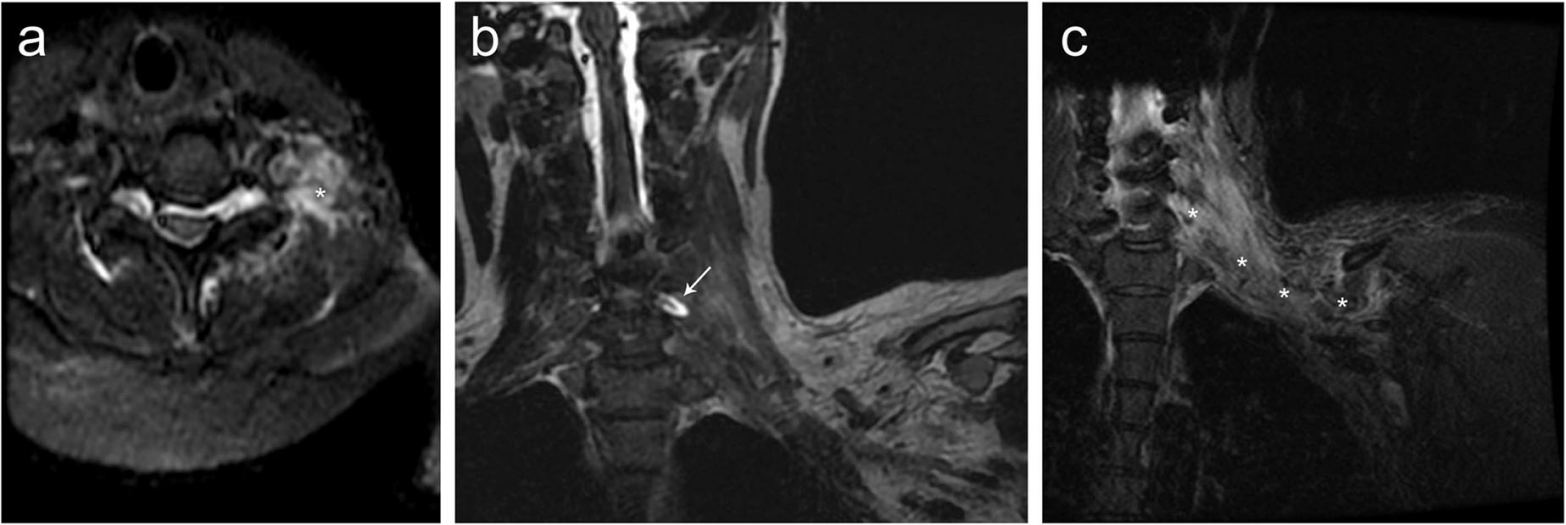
Traction injury of the brachial plexus. Patient with acute traumatic left C7 nerve root avulsion secondary to traction injury of the brachial plexus. Axial (a) short tau inversion recover (STIR) MR images demonstrate increased signal (asterisk) of the left neck base consistent with edema of the soft tissues at the site of injury. Coronal (b) STIR MR image demonstrate a bright signal fluid collection (arrow) which communicates with the cerebral spinal fluid of the spinal cord consistent with posttraumatic pseudomeningocele. Coronal (c) STIR MR image of a companion case demonstrating diffuse increased signal (asterisk) of the left neck base and thoracic inlet consistent with edema due to brachial plexus traction injury
